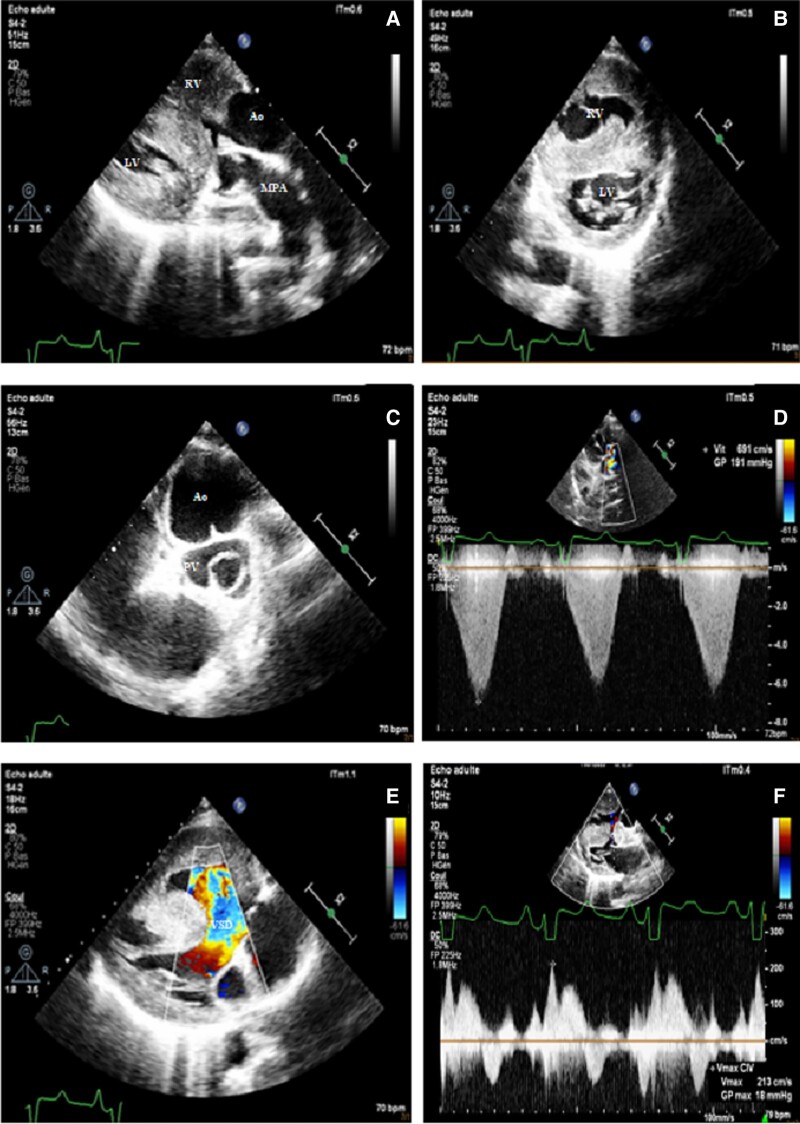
(*A*) TTE parasternal long-axis view demonstrating D-transposition of the great arteries, with the aorta (Ao) originating from the right ventricle (RV) and the main pulmonary artery (MPA) arising from the left ventricle (LV). (*B*) TTE parasternal short-axis view revealing right ventricular (RV) hypertrophy with flattening of the septal curvature in systole. (*C*) TTE parasternal short-axis view showing the tricuspid and anterior-right position of the aortic valve (Ao), and the bicuspid and posterior-left position of the pulmonary valve (PV). (*D*) TTE modified parasternal long-axis view showing multi-level pulmonary obstruction due to sub-pulmonary conus and bicuspid pulmonary valve, with maximum outflow tract velocity at 6.9 m/s. (*E*) TTE parasternal long-axis view demonstrating a large and non-restrictive outlet ventricular septal defect (VSD) causing right-to-left shunting in diastole. (*F*) TTE parasternal long-axis view showing a large and non-restrictive outlet VSD causing left-to-right shunting in systole (max velocity: 2.1 m/s).
